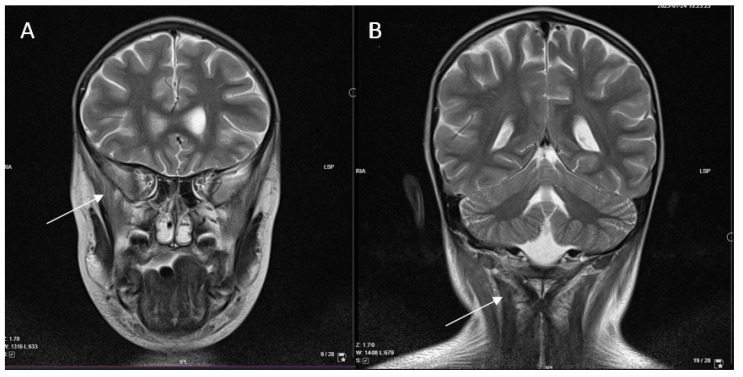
Head MRI, T2 sequence. Diffuse high signal in the *m. temporalis* (A) and neck muscles (B), as well as uneven accumulation of contrast medium in the *m. semispinalis* showing muscle oedema.
